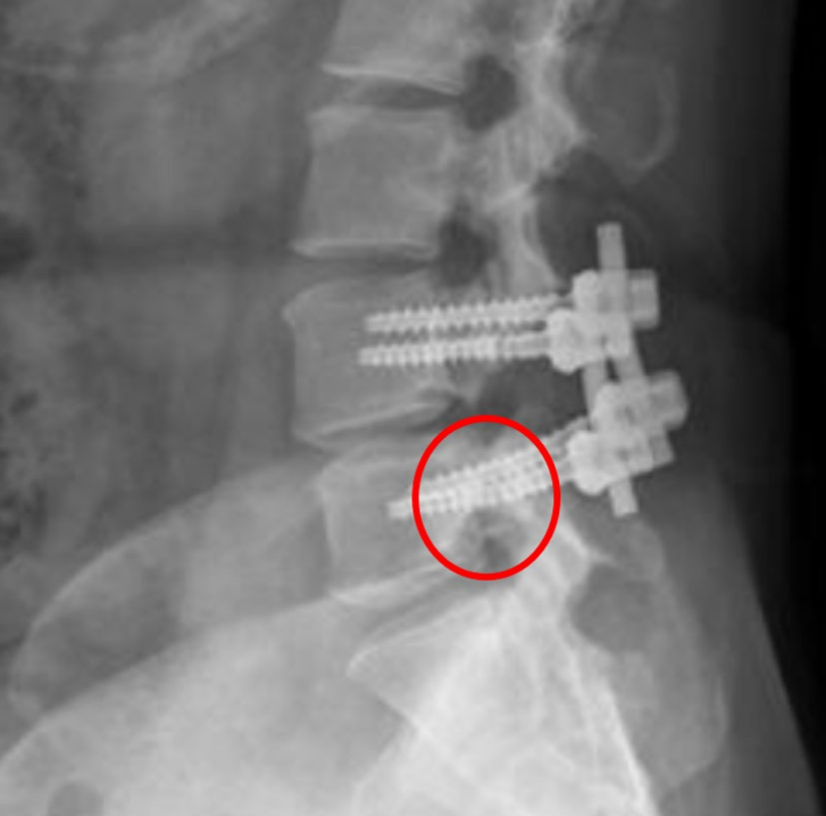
Radiographic of screw: radiographic examination showed the screw breakage (*red circle*)
